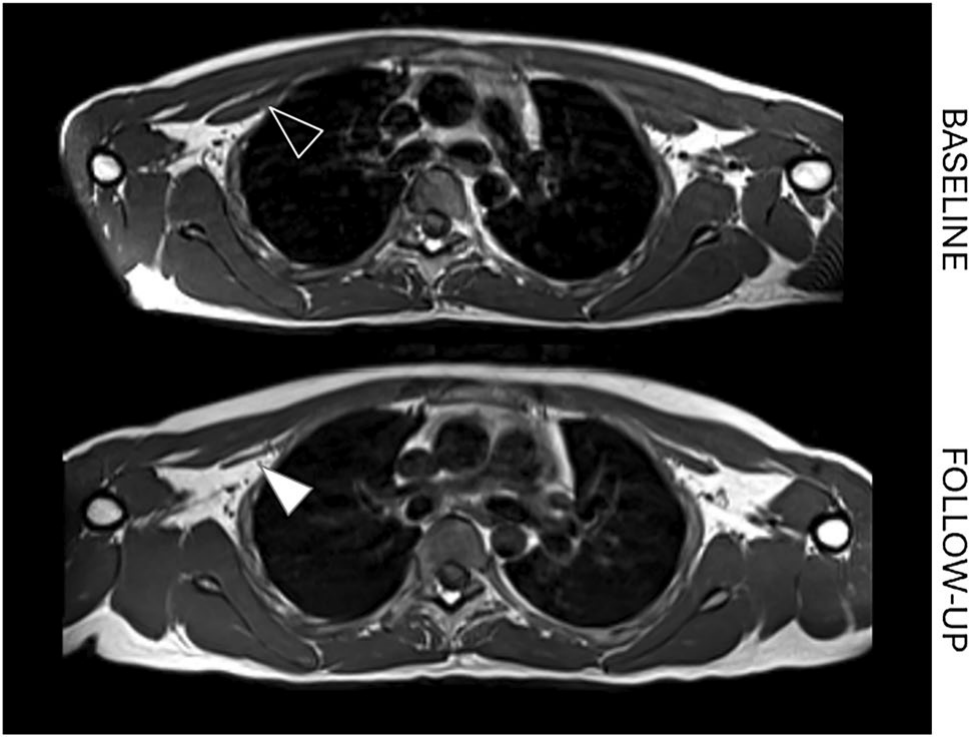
Atrophy progression. STIR-independent atrophy-progression of pectoralis minor at first (up) and last (down) MRI study after 37 months (P6, 20 years-old male, FU duration 37, months, MIRS 3, E2 expansion class)
